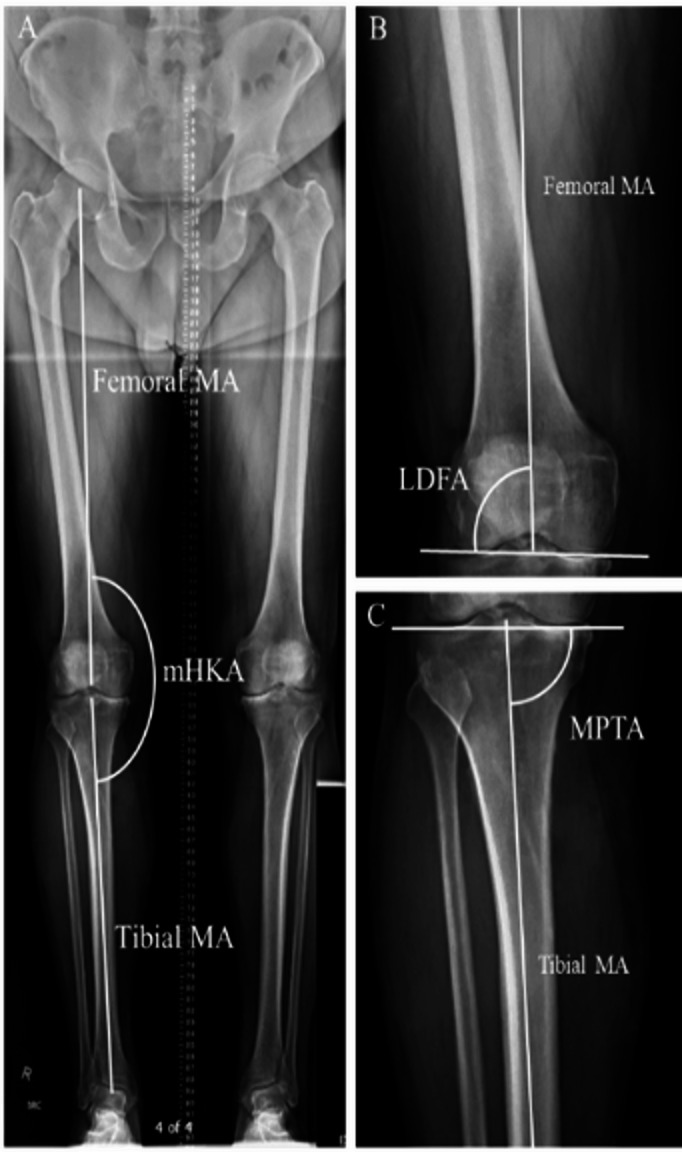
Coronal alignment parameters measured on long-leg radiographs. A Mechanical hip–knee–ankle angle (mHKA), defined as the angle between the femoral and tibial mechanical axes. B Lateral distal femoral angle (LDFA), defined as the angle between the femoral mechanical axis and the distal femoral joint line. C Medial proximal tibial angle (MPTA), defined as the angle between the tibial mechanical axis and the proximal tibial joint line. MA, mechanical axis
